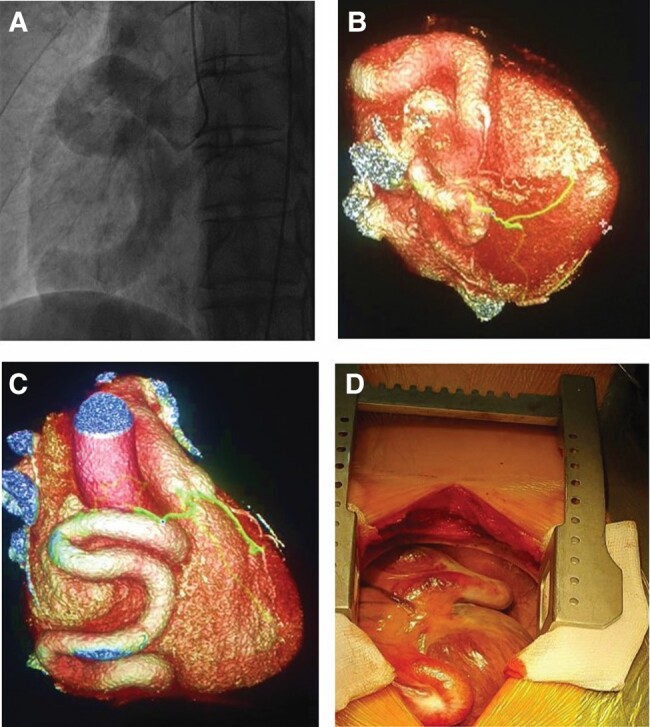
(*A*) RCA visualized on invasive coronary angiogram. (*B* and *C*) 3D reconstruction of the aneurysmal RCA on CT. (*D*) Intraoperative view of the aneurysmal RCA.
